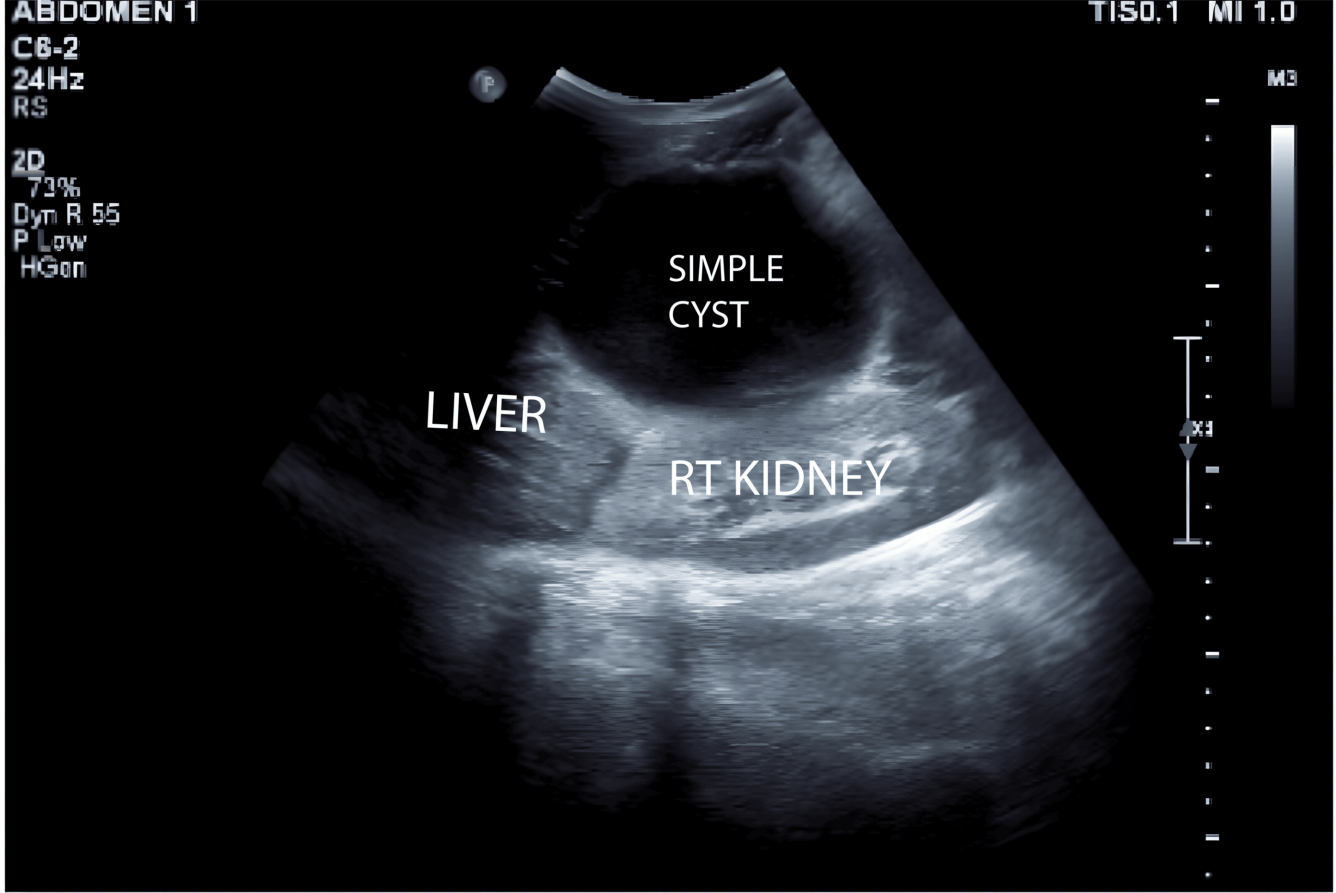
Ultrasound of the abdomen - image 2 The image shows a simple anechoic cyst indenting the right lobe of the liver (superiorly) and compressing the right kidney (posteriorly)
